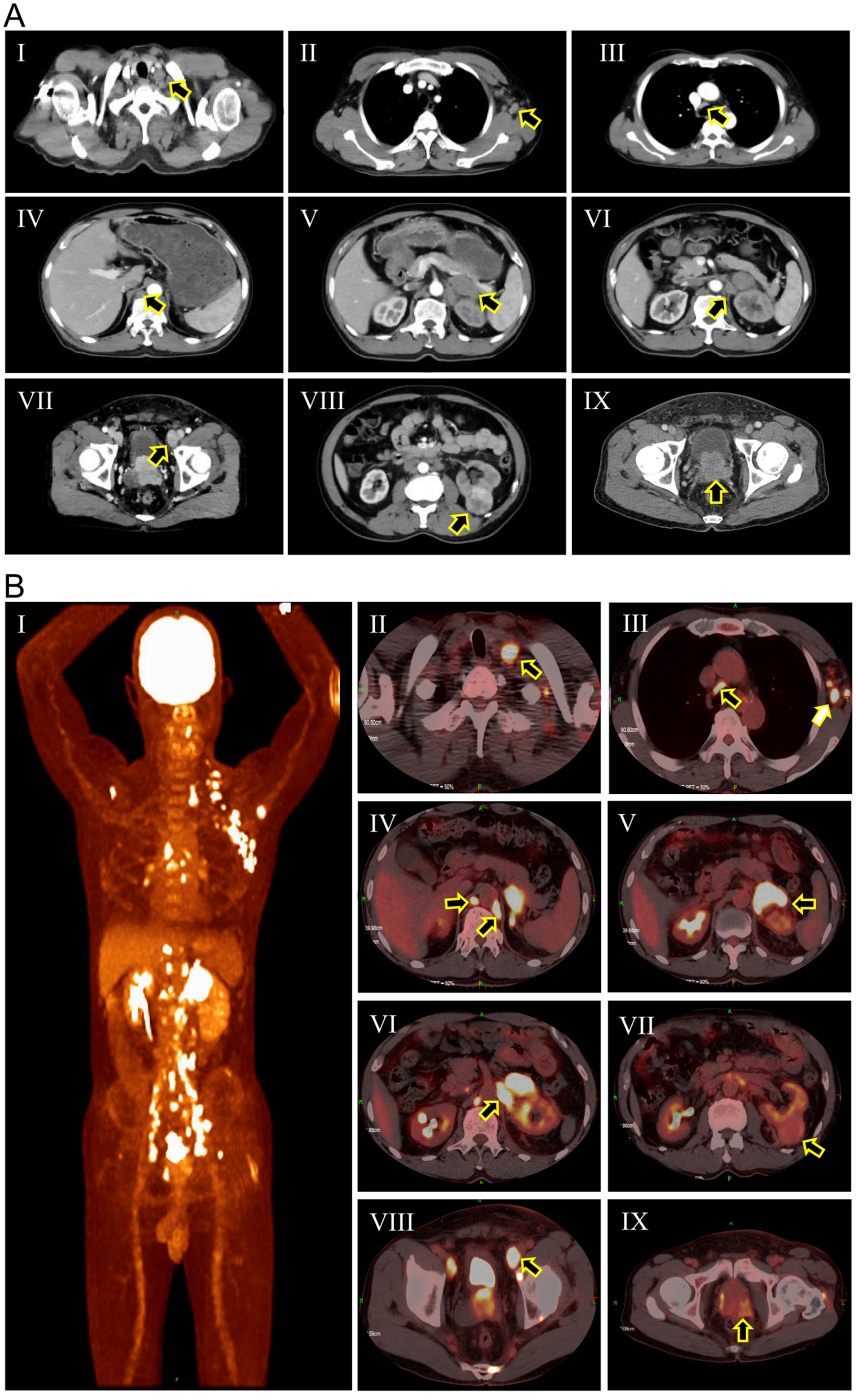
Computed tomography scan (A) and ^18^F-FDG PET/CT scan (B) of the patient at initial diagnosis. (A-I) Cervical lymph nodes (black arrow); (A-II) Axillary lymph nodes (black arrow); (A-III) Mediastinal lymph nodes (black arrow); (A-IV) Retroperitoneal lymph nodes (black arrow); (A-V) Adrenal metastasis (black arrow); (A-VI) Renal hilar lymph nodes (black arrow); (A-VII) External iliac lymph nodes (black arrow); (A-VIII) Renal mass (black arrow); (A-IX) Enlarged prostate; (B-I) Overview of pathological uptake; (B-II) Cervical lymph nodes (black arrow); (B-III) Axillary lymph nodes (white arrow) and mediastinal lymph nodes (black arrow); (B-IV) Retroperitoneal lymph nodes (black arrows). (B-V) Adrenal metastasis (black arrow); (B-VI) Renal hilar lymph nodes (black arrow); (B-VII) Renal mass (black arrow); (B-VIII) External iliac lymph nodes (black arrow); (B-IX) Prostatic mass (black arrow).
